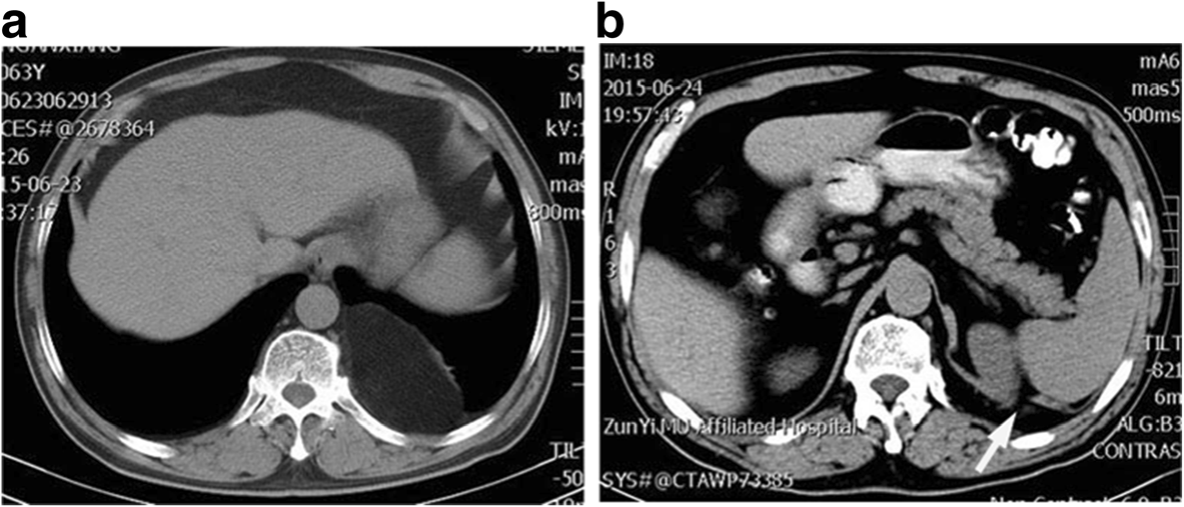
Preoperative chest and abdominal computed tomography (CT) scans. The chest CT shows a posterolateral left-sided mass with fat tissue density (a), and the *white arrow* indicates the diaphragmatic defect (b)
